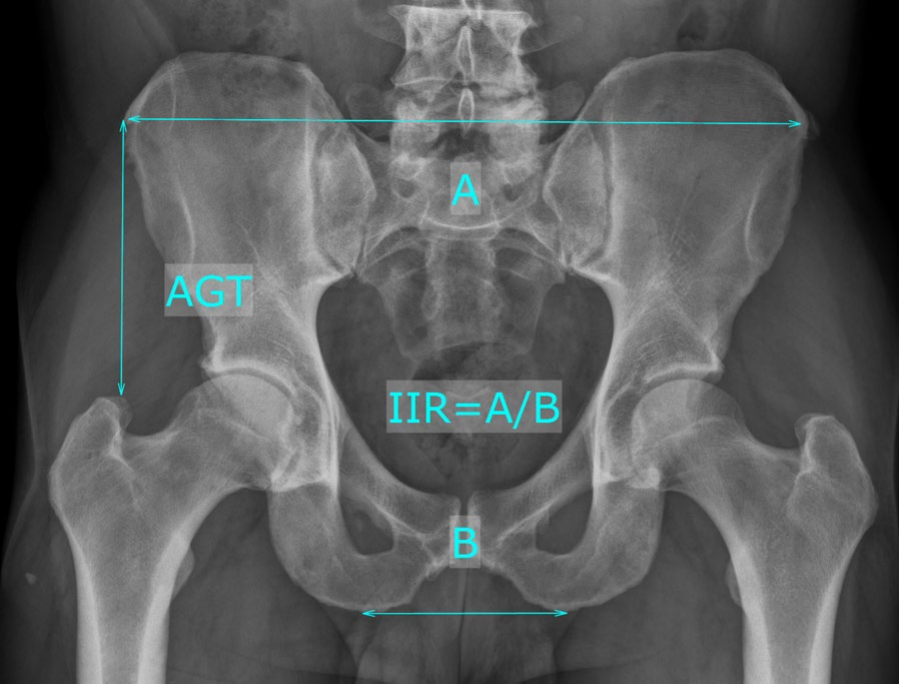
Pelvic morphological parameters on an anteroposterior pelvic radiograph: ilium–ischial ratio (IIR) = A/B; distance anterior superior iliac spine to the tip of the greater trochanter (AGT)
